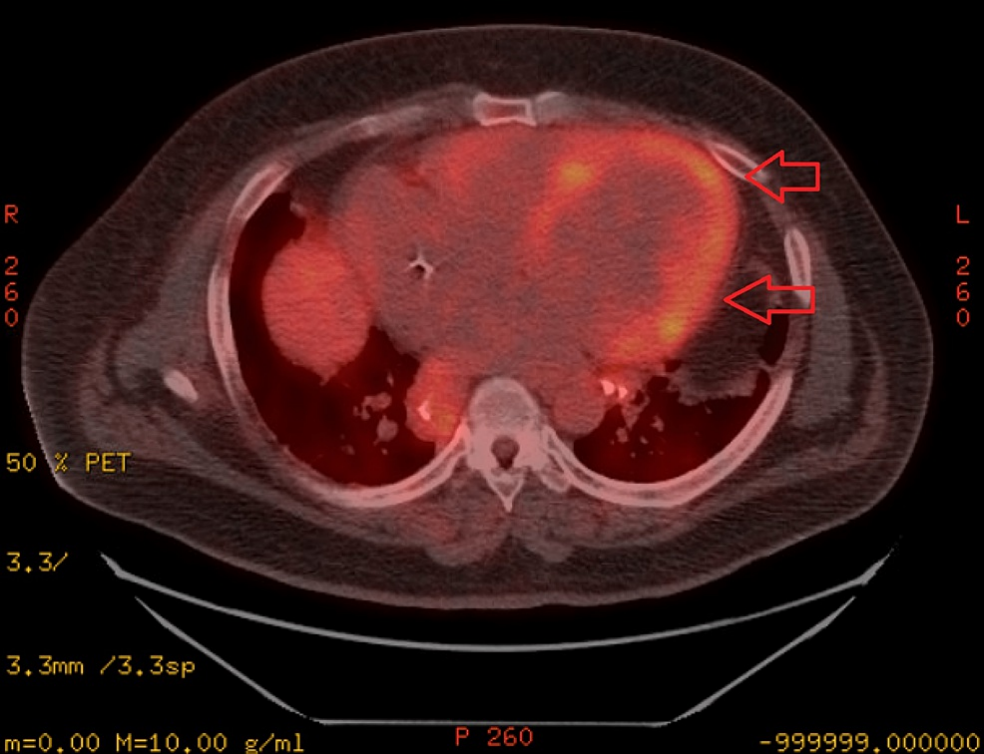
PET scan axial view revealing diffuse FDG uptake, most prominent in the anterior, anterolateral, and inferior wall, consistent with diffuse active sarcoidosis PET: positron emission tomography; FDG: fluorodeoxyglucose
